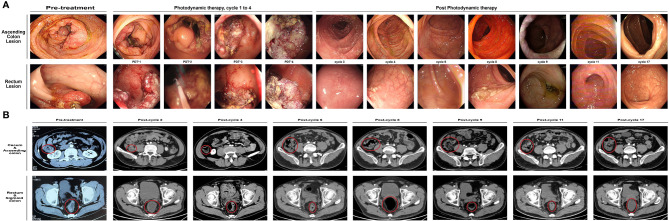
Visualizations of treatment efficacy in this patient. (A) Tumor lesions in the ascending colon and the rectum under coloscopy during the treatment. All lesions showed significant necrotic changes with photodynamic therapy. After the local photodynamic therapy, no tumor residual and local recurrence were observed under coloscopy through these cycles. (B) The patient’s abdominal CT scan images in cecum/ascending colon and rectum (indicated by red circles) pre-treatment, post cycle-2, post-cycle-4, post cycle-6, post cycle-8, post cycle-9, post cycle-11 and post cycle-17, respectively.
